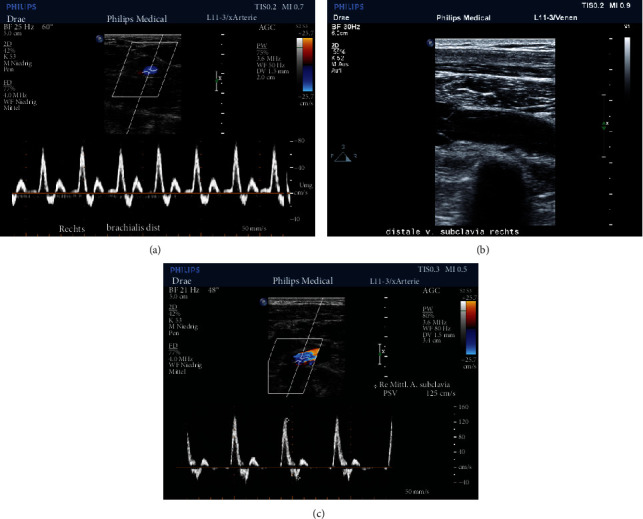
(a–c) Sonography Philips LX 50 transducer L12-3, 12-3 MHz, 160 elements. (a) Brachial artery and vein showing a normal flow signal. (b) Subclavian vein without signs of thrombosis, a venous valve on the left side visible, and no signs of external compression. (c) Subclavian artery and vein. Normal arterial flow, patent vein with no signs of thrombus or external compression.
